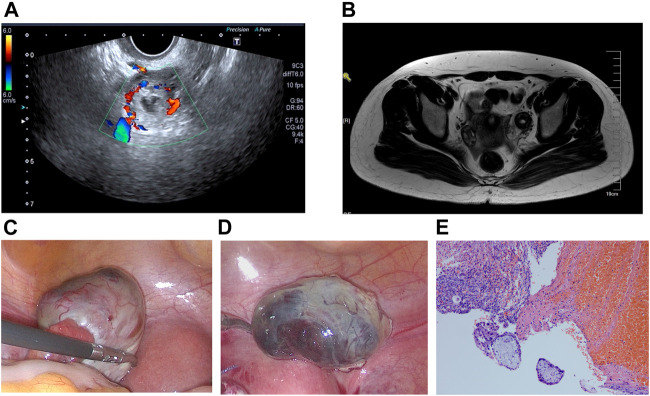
(A) Transvaginal ultrasonography revealed an echogenic mass in the left ovary. (B) MRI diagnosis revealed a left ovarian mass. (C) Ovarian pregnancy was detected using a laparoscope. (D) Ovarian pregnancy lesion was completely removed. (E) Postoperative pathological analysis confirmed left ovarian gestation, revealing both chorionic villi and trophoblasts.
